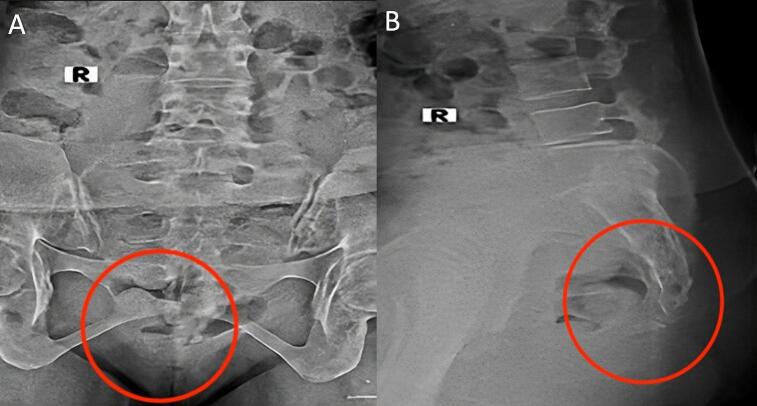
The X-ray examination before surgery showed a sacrococcygeal fracture in AP view (a) and lateral view (b).
